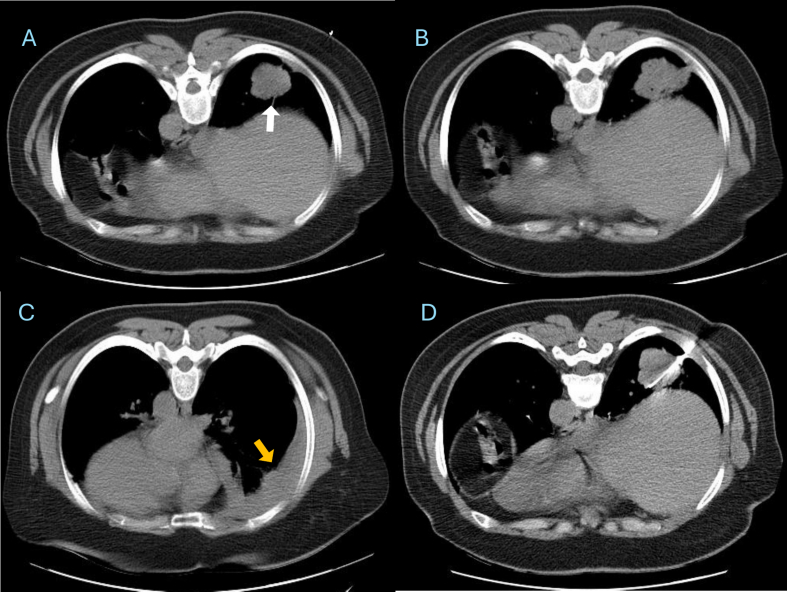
Axial CT images in the prone position showing a peripheral right lower-lobe lung mass (white arrow) immediately before and during CT-guided core needle biopsy. A minimal right pleural effusion is visible (yellow arrow). (For interpretation of the references to colour in this figure legend, the reader is referred to the web version of this article.)
